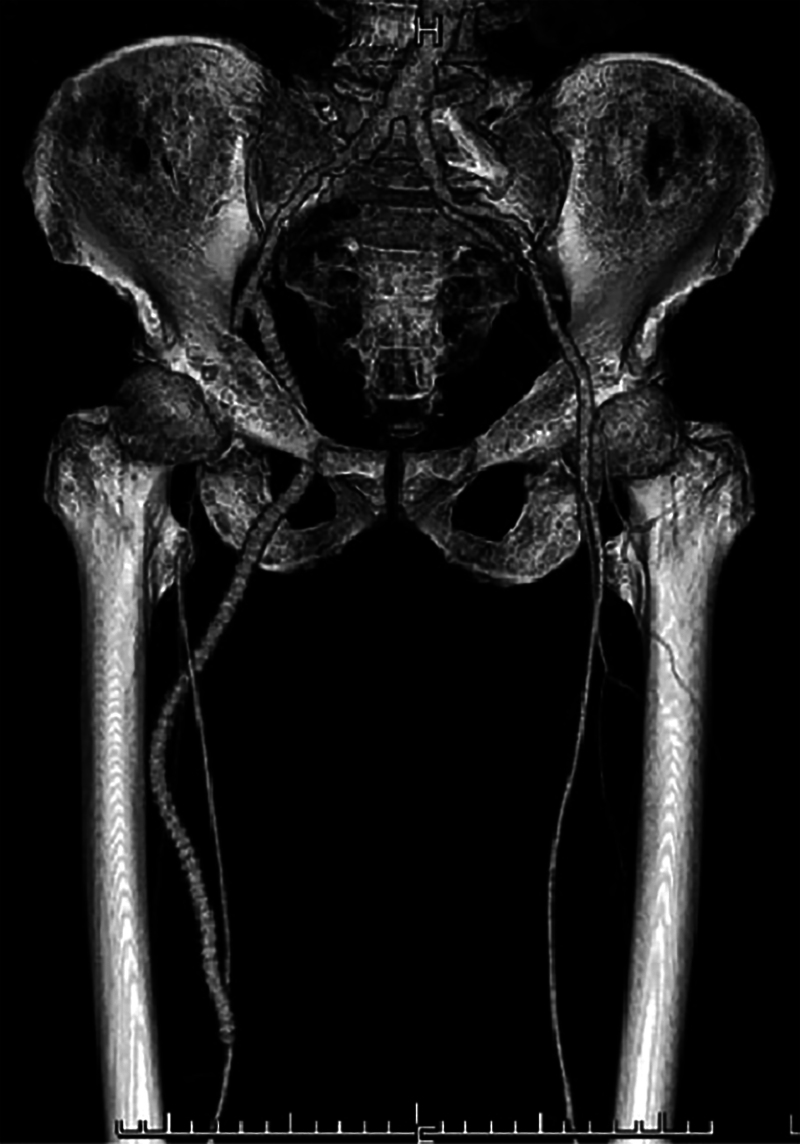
Computed tomography angiogram of right obturator bypass
